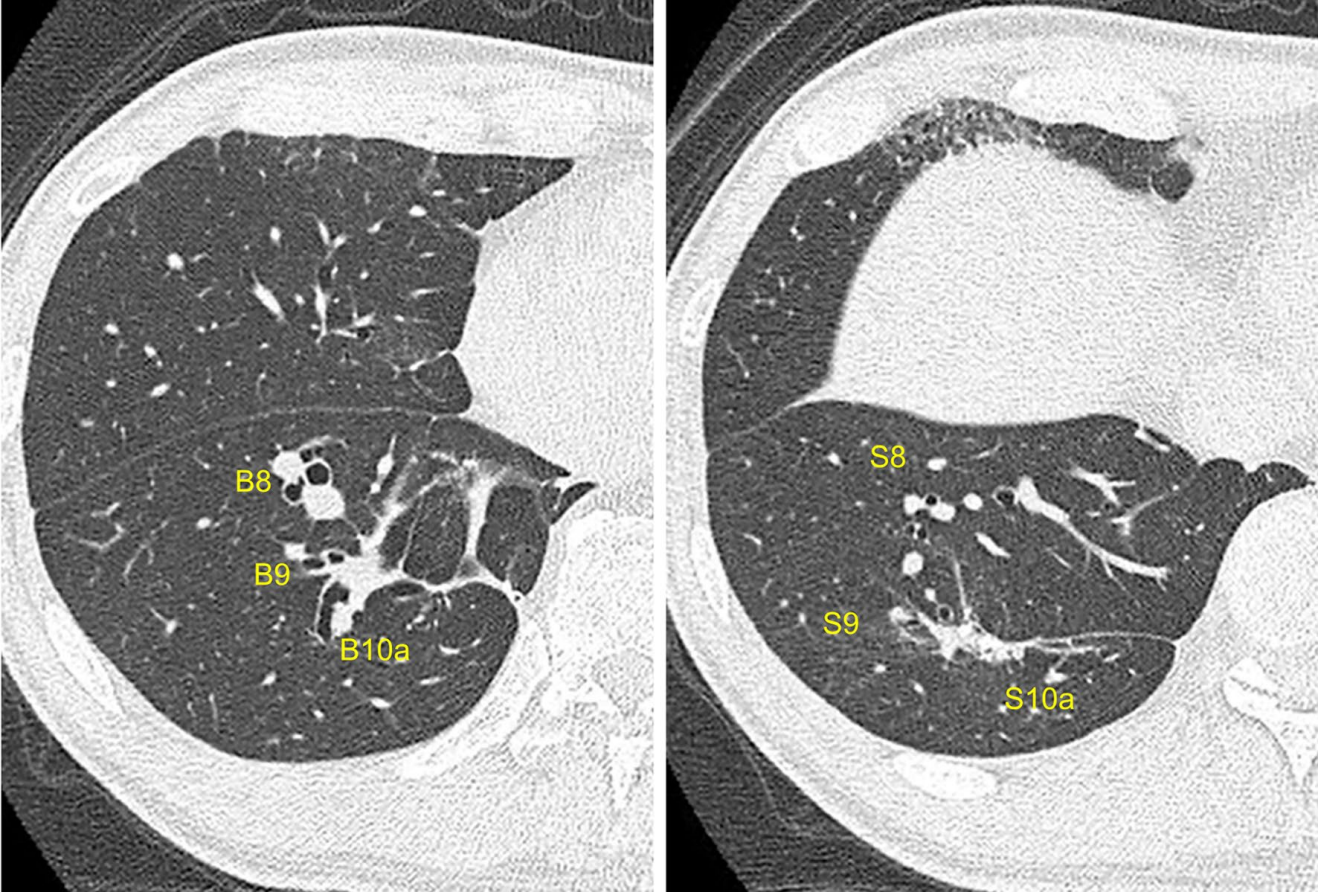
Postoperative image of computed tomography
